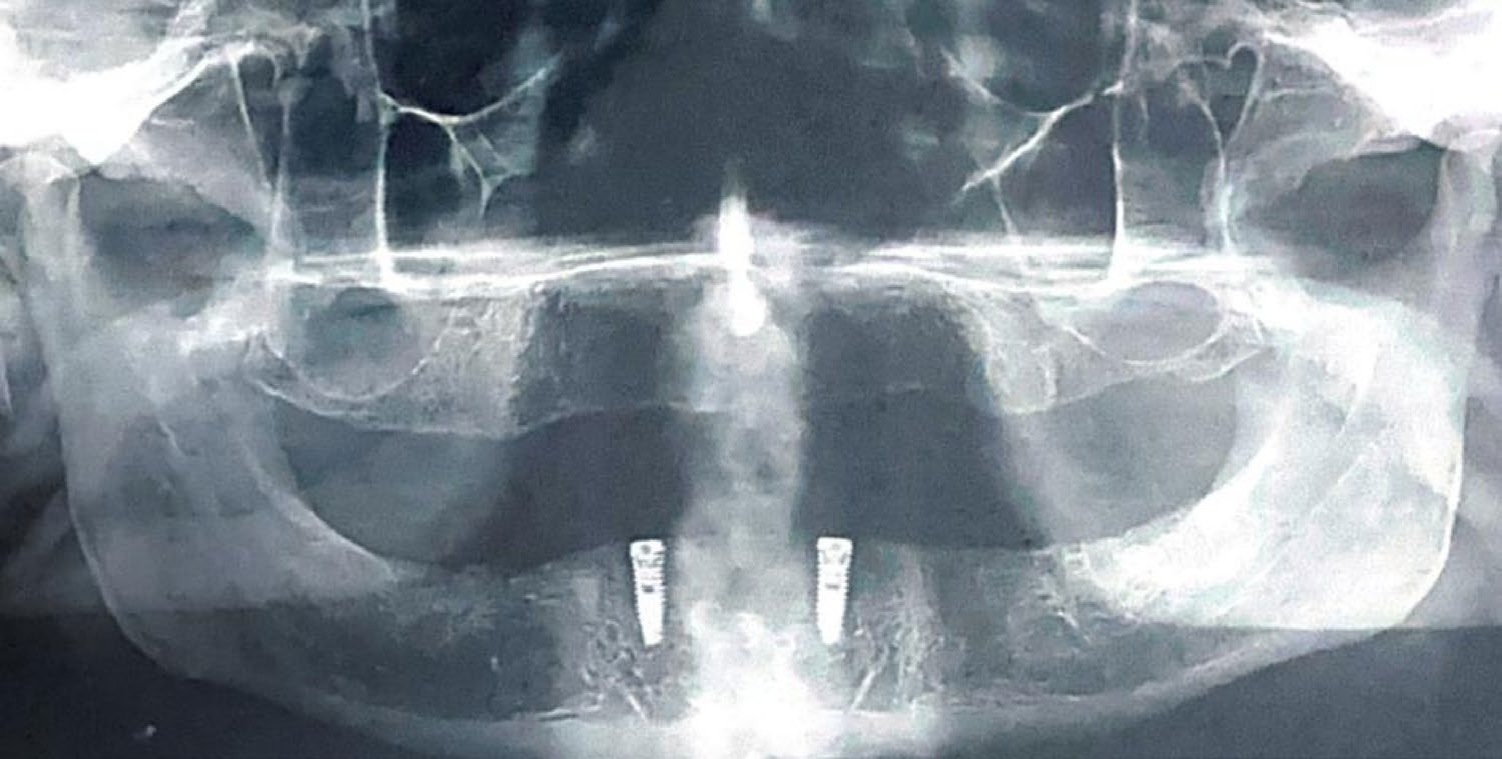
Panoramic x-ray for verification of orientation and position of implants.
